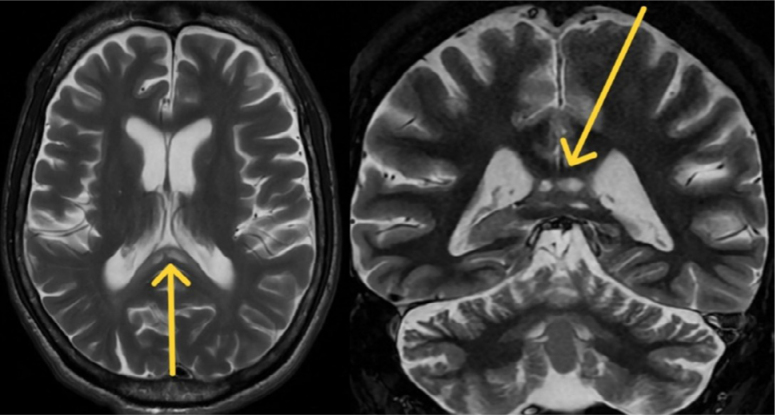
Brain magnetic resonance imaging axial and coronal T2 sequences showing hyperintense lesions, without mass effect, involving the splenium of the corpus callosum.
